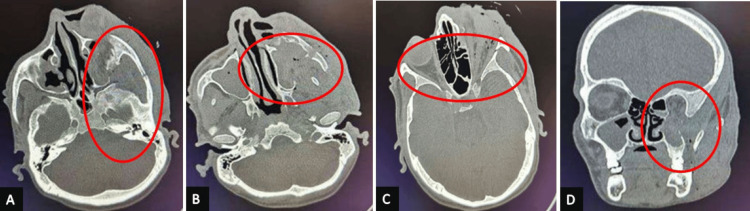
Intraoperative views on the computed tomography (A) Fracture of the left zygomatic arch, infraorbital rim, and avulsion of the left eye. (B) Fracture of the anterior and posterolateral wall of the maxillary sinus. (C) Separation of the left frontozygomatic suture. (D) Fracture of the left medial wall and floor of the orbit
